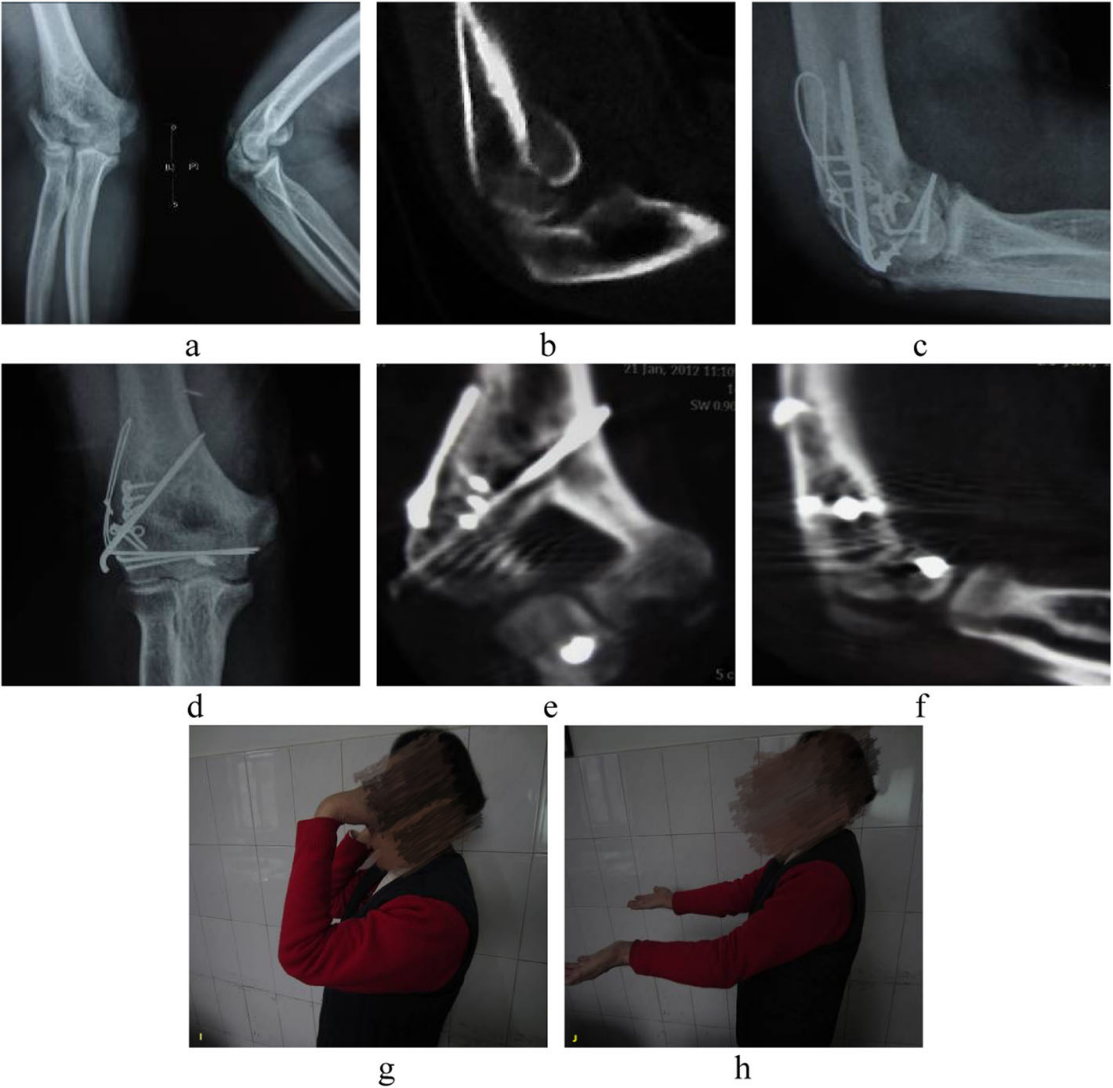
Female, 62 years old, preoperative anteroposterior and lateral radiographs of capitellum and trochlea fractures caused by falling injury from stairs (a); preoperative CT showing the capitellum and trochlea fractures and intact posterior condyle (b); X-ray radiographs (c, d) and CT scans (e, f) 2 days after operation showing the reduction and fixation of the capitellum and trochlea , as well as the good restoration of articular surface of the distal end; functional appearance 1 month after operation showing satisfactory elbow function (g, h)
